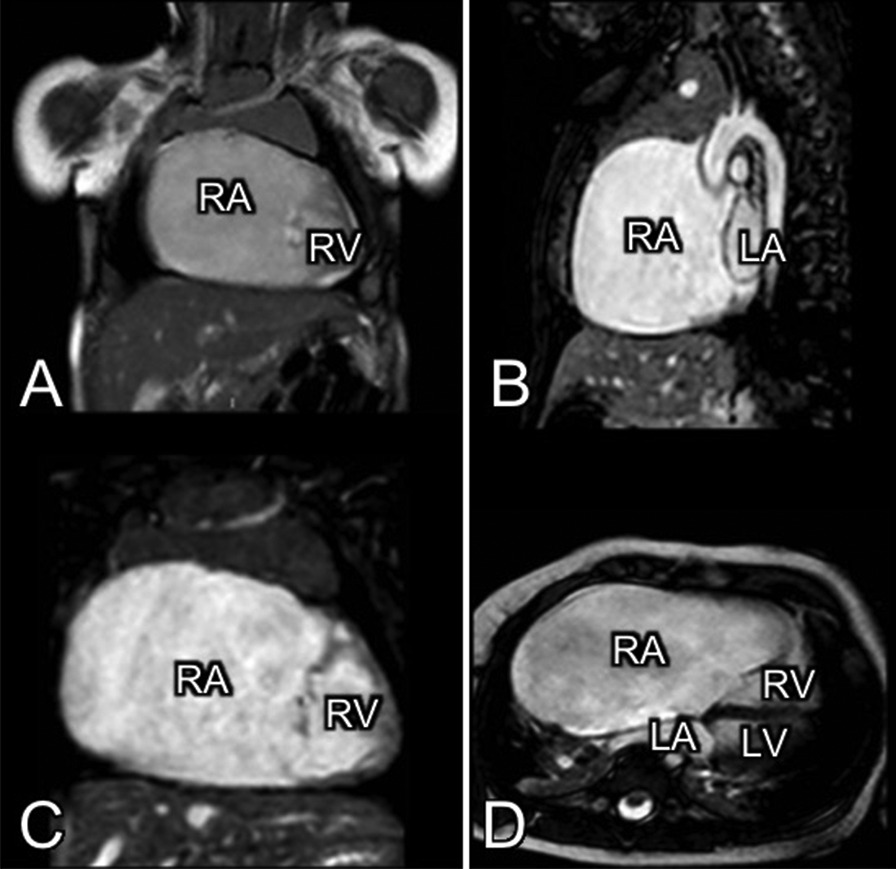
MRI at 6 months. Cardiomegaly with a normal size of the right ventricle. A, C show a coronal plane, B shows a sagittal plane and D shows an axial plane. *RA: Right atrium; RV: Right ventricle; LA: Left atrium: LV: Left ventricle*
